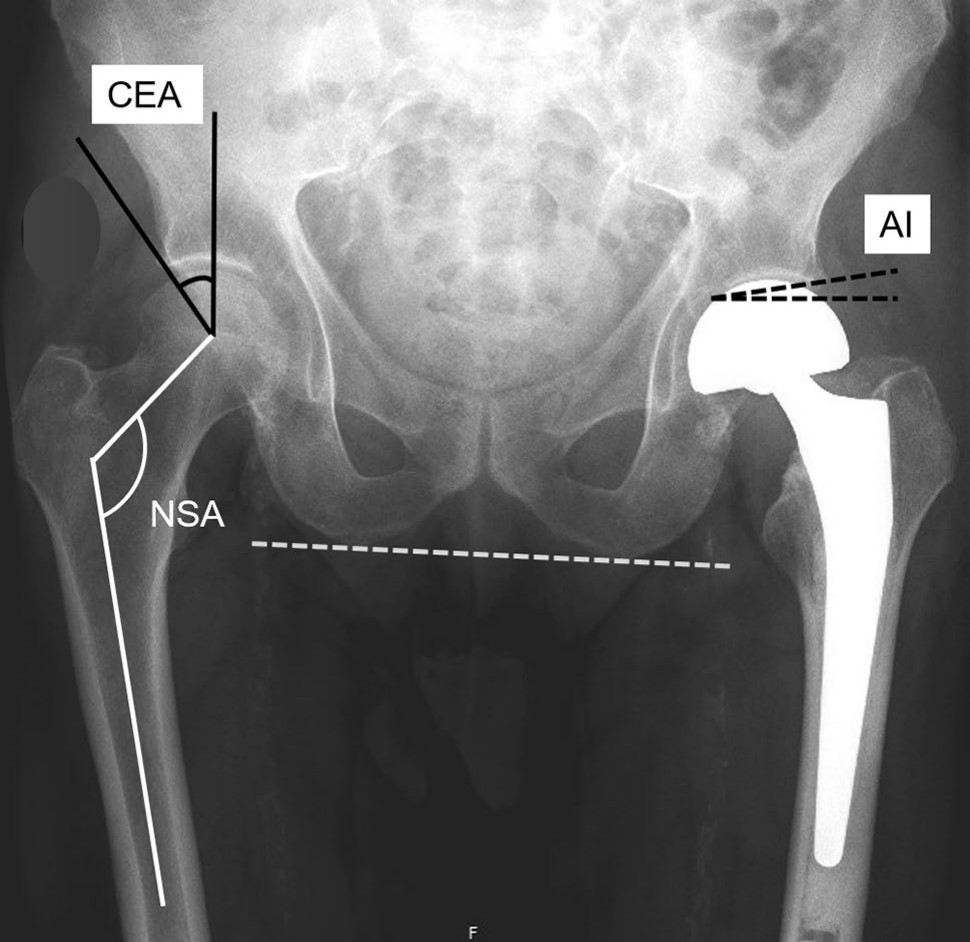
Standard pelvic radiograph with delineated acetabular center edge angle (CEA), neck-shaft angle (NSA) and acetabular index (AI)
